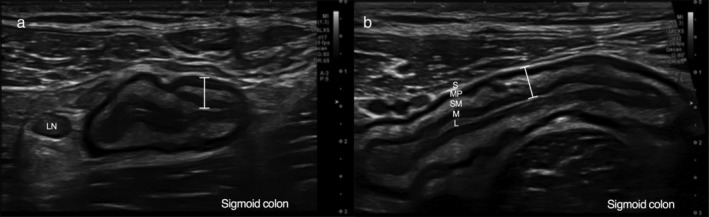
Colonic inflammation detected using point‐of‐care gastrointestinal ultrasound. (a) Transverse section of inflamed sigmoid colon in a patient with Crohn's colitis characterized by increased wall thickness (5.5 mm, measured using marker), abnormal wall stratification with submucosal prominence, and an enlarged lymph node within mesenteric hyperechogenicity reflective of fibrofatty proliferation. Note that Doppler signal is not shown. (b) Longitudinal section of same inflamed sigmoid colon in a patient with Crohn's colitis with a marker again showing increased wall thickness (5.5 mm). The bowel wall layers are annotated: L, luminal interface, white; M, mucosa, black; SM, submucosa, white; MP, muscularis propria, black; S, serosa, white. Note that Doppler signal is not shown.
